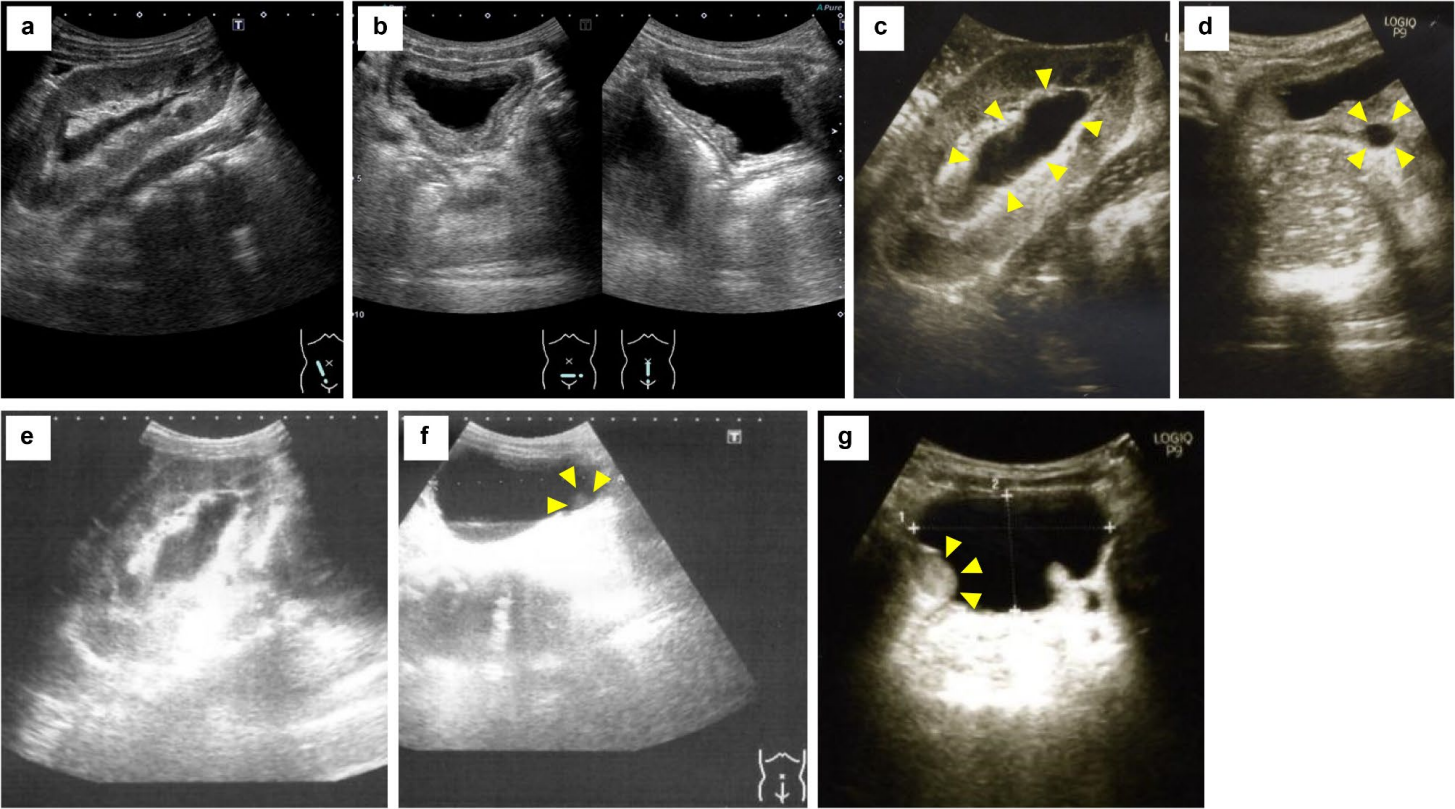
Ultrasonography findings at 4 months after kidney transplantation (KT) and after Deflux^®^ injection treatment. At 4 months after KT, ultrasonography shows mild hydronephrosis of the transplanted kidney (Society of Fetal Urology [SFU] grade 1) (a). No dilation of the distal ureter is observed, and the bladder wall is thickened (b). Ultrasonography immediately after surgery shows that the renal pelvis is more dilated than it was preoperatively (c). Ureteral dilatation is observed (yellow arrowhead) (d). (e and f) Postoperative day 1. After Deflux^®^ injection treatment, hydronephrosis of the transplanted kidney remains unchanged (SFU grade 1) (e). A bulge associated with Deflux^®^ is observed at a location consistent with the injection site (yellow arrowhead) (f). Postoperative day 3. After Deflux^®^ injection treatment, a bulge associated with Deflux^®^ at a location consistent with the injection site is observed (yellow arrowhead) (g)
